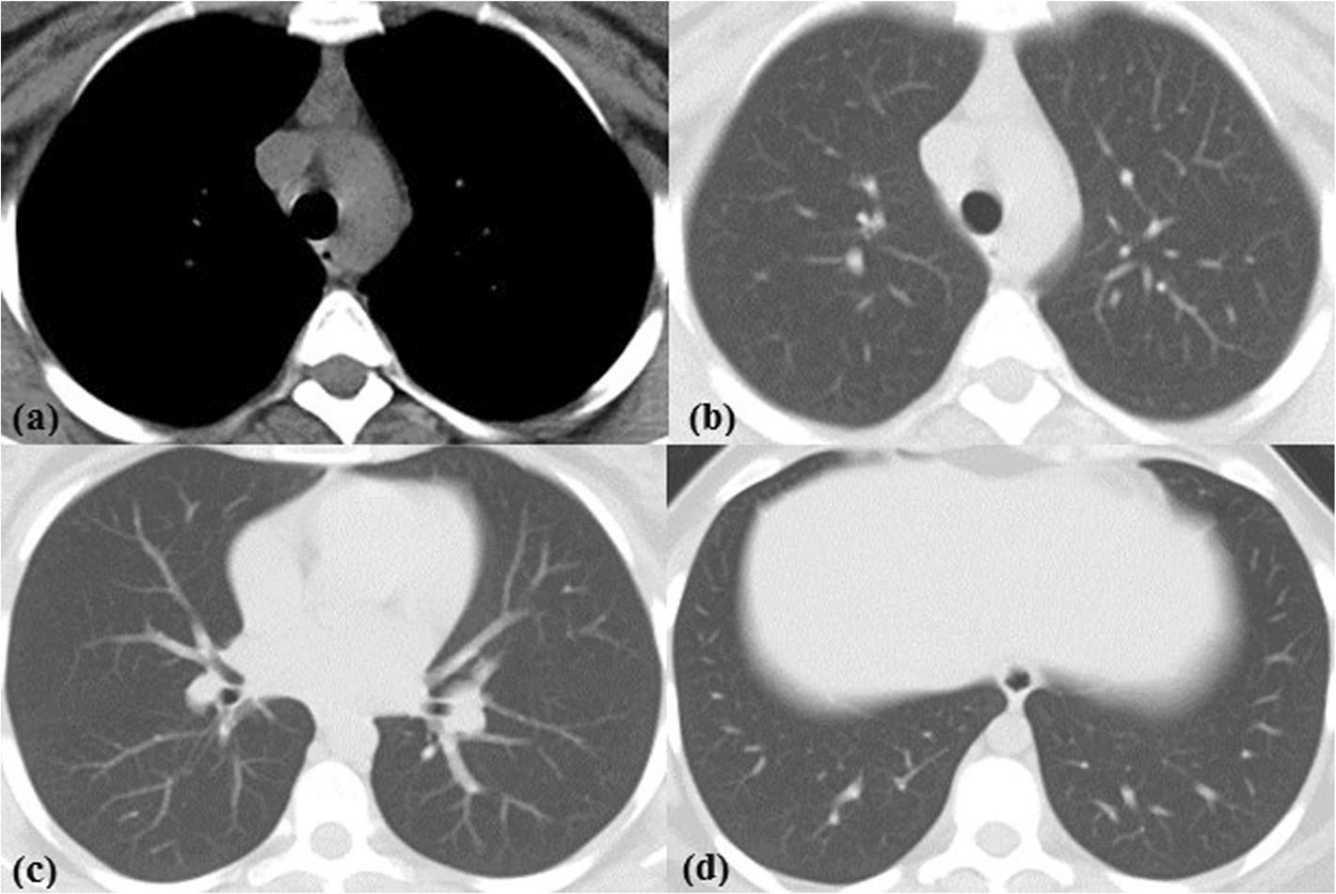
Thymic hyperplasia in a 23-year-old female with RT-PCR proved COVID-19. a Non-contrast CT chest axial cut mediastinal window shows retrosternal well-defined triangular-shaped soft tissue density with straight borders with less macroscopic fat than expected for age. b–d Axial lung window showing no lung abnormality
